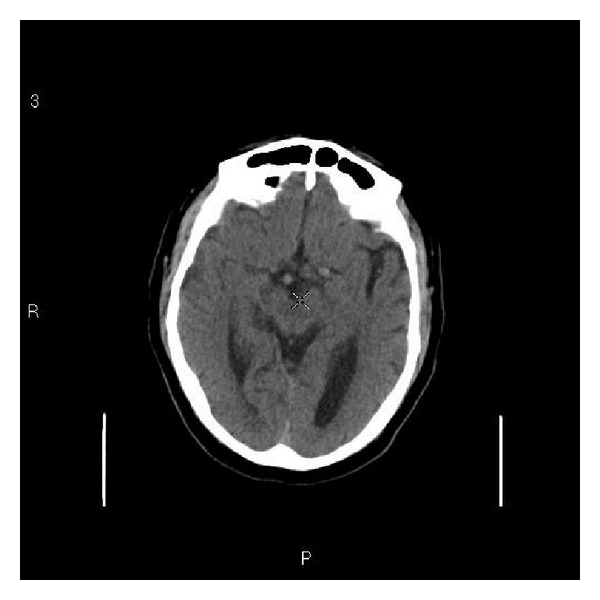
Noncontrast CT scan showing age related atrophy changes.
